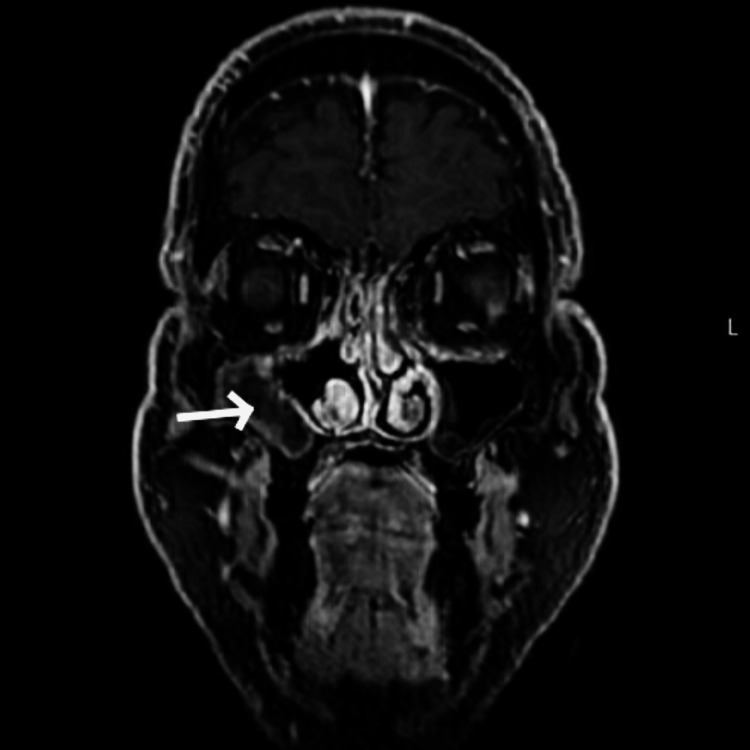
MRI after endoscopic sinus surgery of right maxillary sinus (white arrow). Coronal contrast-enhanced MRI T1 fat-saturated image at the level of maxillary sinus after surgical treatment, showing middle meatal antrostomy and removal of the enhancing lesion, with post-surgical findings with no signs of residual lesions (white arrow). Right-handed side.
